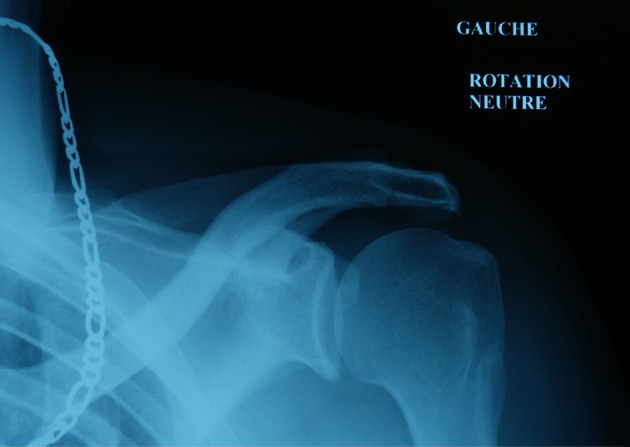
X-ray hypodense lesion in the cortical bone.
